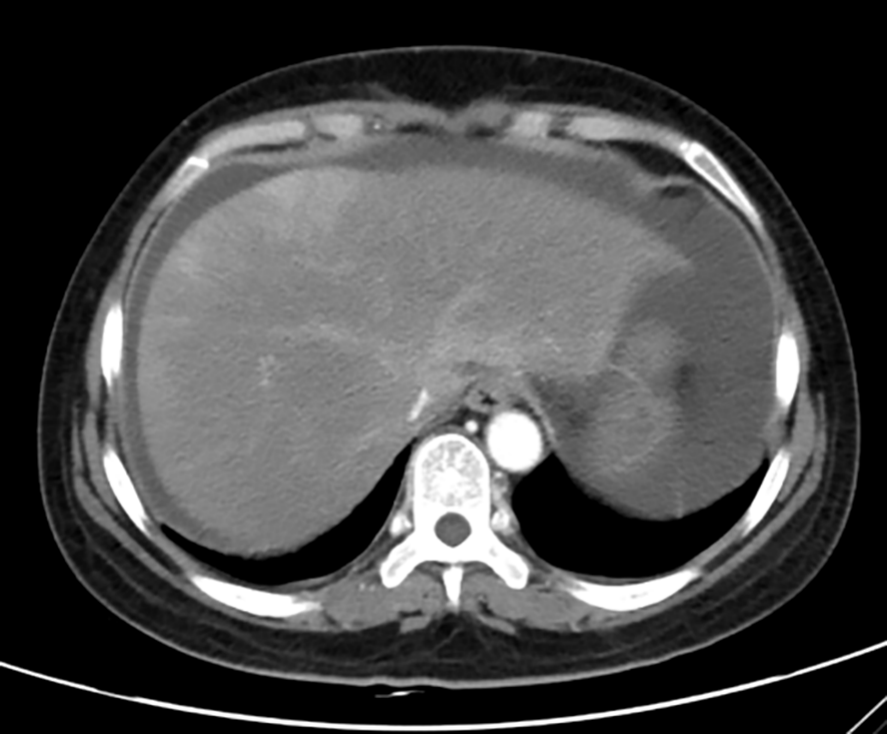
Enhanced CT scan of liver (Decreased and uneven density of liver parenchyma, increased abdominal and pelvic effusion, thus, drug-induced liver injury was considered).
